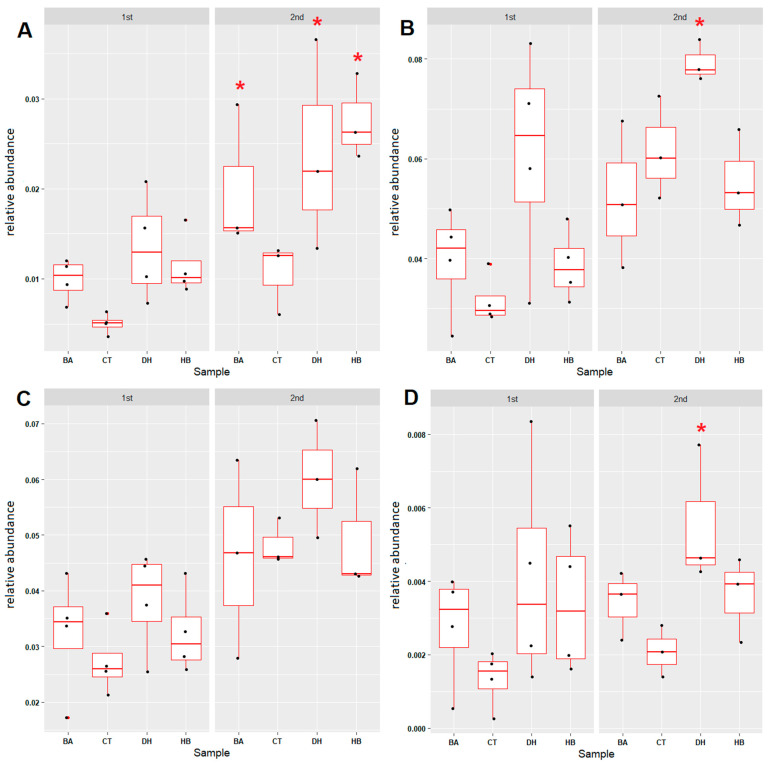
Overall relative abundance changes in sulfur-cycle-associated microbial communities in the BA-, HB- and DHA-treated river sediments. (A) Thiosulfate oxidation (M00595: thiosulfate => sulfate). (B) Assimilatory sulfate reduction (M00176: sulfate => H_2_S). (C) Sulfate-sulfur assimilation (M00616). (D) Dissimilatory sulfate reduction (M00596: sulfate => H_2_S). The term “1st” indicates the period between the 0th week to the 8th week; “2nd” indicates the period between the 8th week to the 17th week. Red stars indicate the *p*-value of the Mann–Whitney U test < 0.05 (compared with control (CT)). CT: control; BA: benzoic acid; HB: 4-hydroxybenzoate; DH: dehydroacetic acid.
